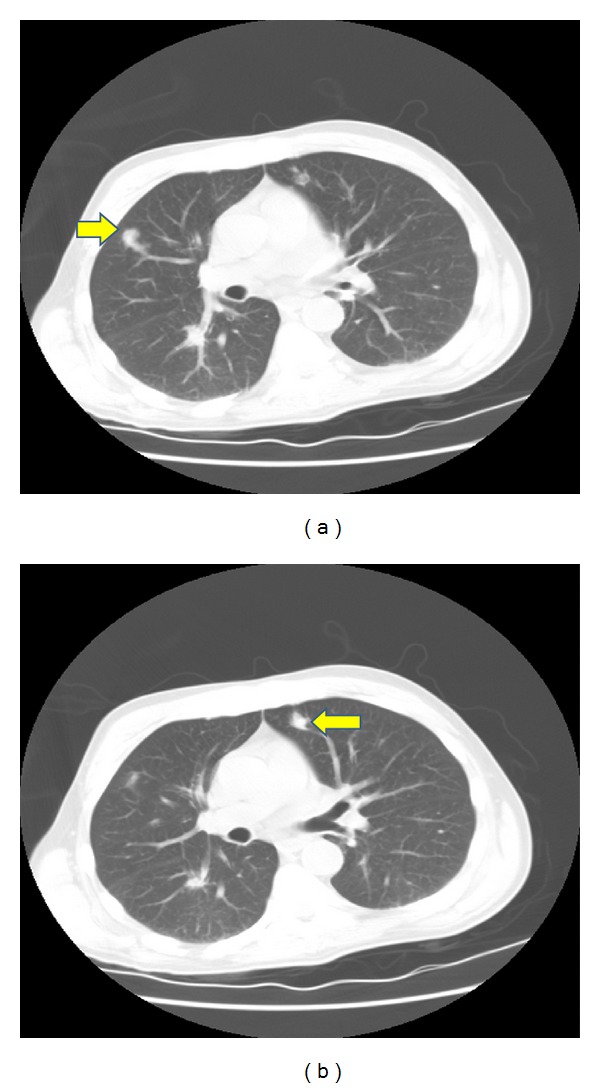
CT chest showing the pulmonary nodules.
